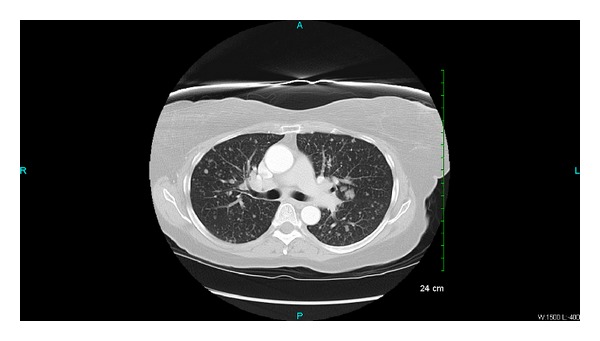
Chest CT scan showing multiple diffuse noncalcified nodules in both lung fields consistent with metastases.
